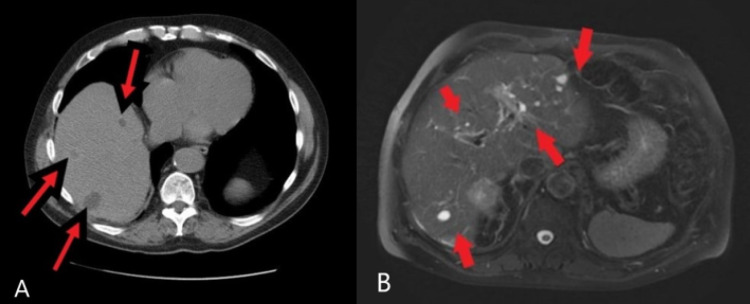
Hepatic metastases and subcapsular metastases on CT and MRI. (A) Preoperative CT scan, axial view of vague hypodensities in segments six and seven in the liver which are not dense enough to represent cysts. Concerning for metastatic disease (red arrow). (B) Preoperative MRI of the liver, innumerable cysts, multiple hypoenhancing and hyperenhancing lesions read as hepatic metastases, as well as subcapsular metastases (red arrow). CT: computerized tomography; MRI: magnetic resonance imaging
